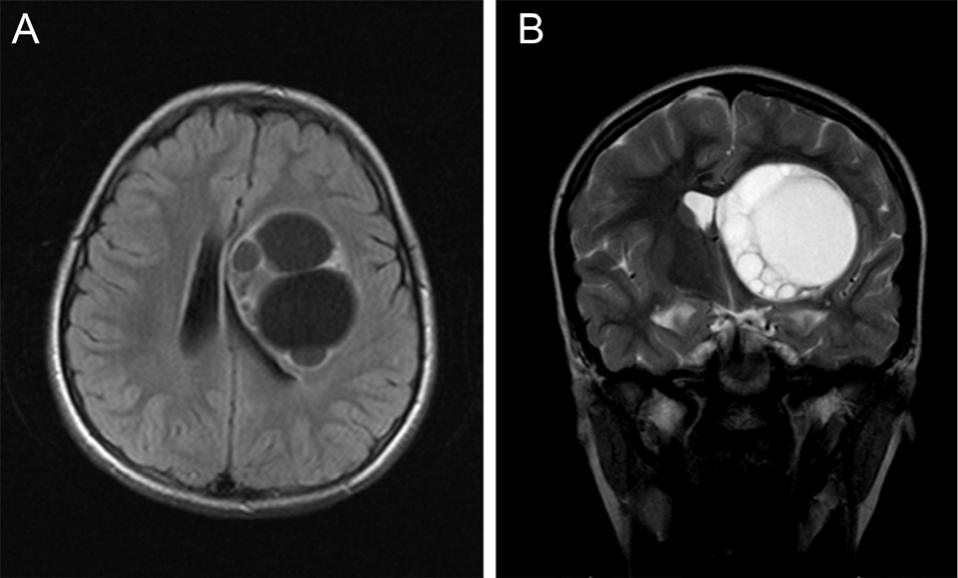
Axial FLAIR (A) and coronal T2-weighted (B) magnetic resonance image of the brain demonstrating a well-defined T2 hyperintense lesion that is totally suppressed on the FLAIR sequence, with no sign of perilesional edema.
